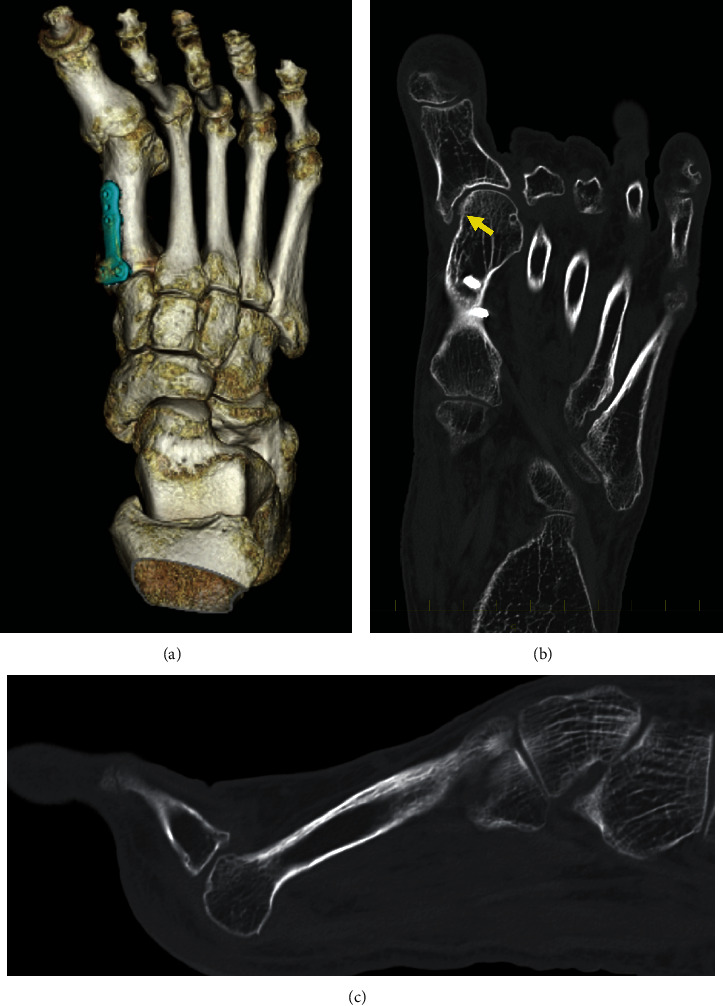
Preoperative CT images. (a) 3DCT image. (b) Coronal image. Arrow indicates decreasing the surface area of the medial side of the articular surface at the first metatarsal head by resection of the bony prominence. (c) Dorsal dislocation of the 2nd toe. CT: computed tomography; 3DCT: three-dimensional computed tomography.
